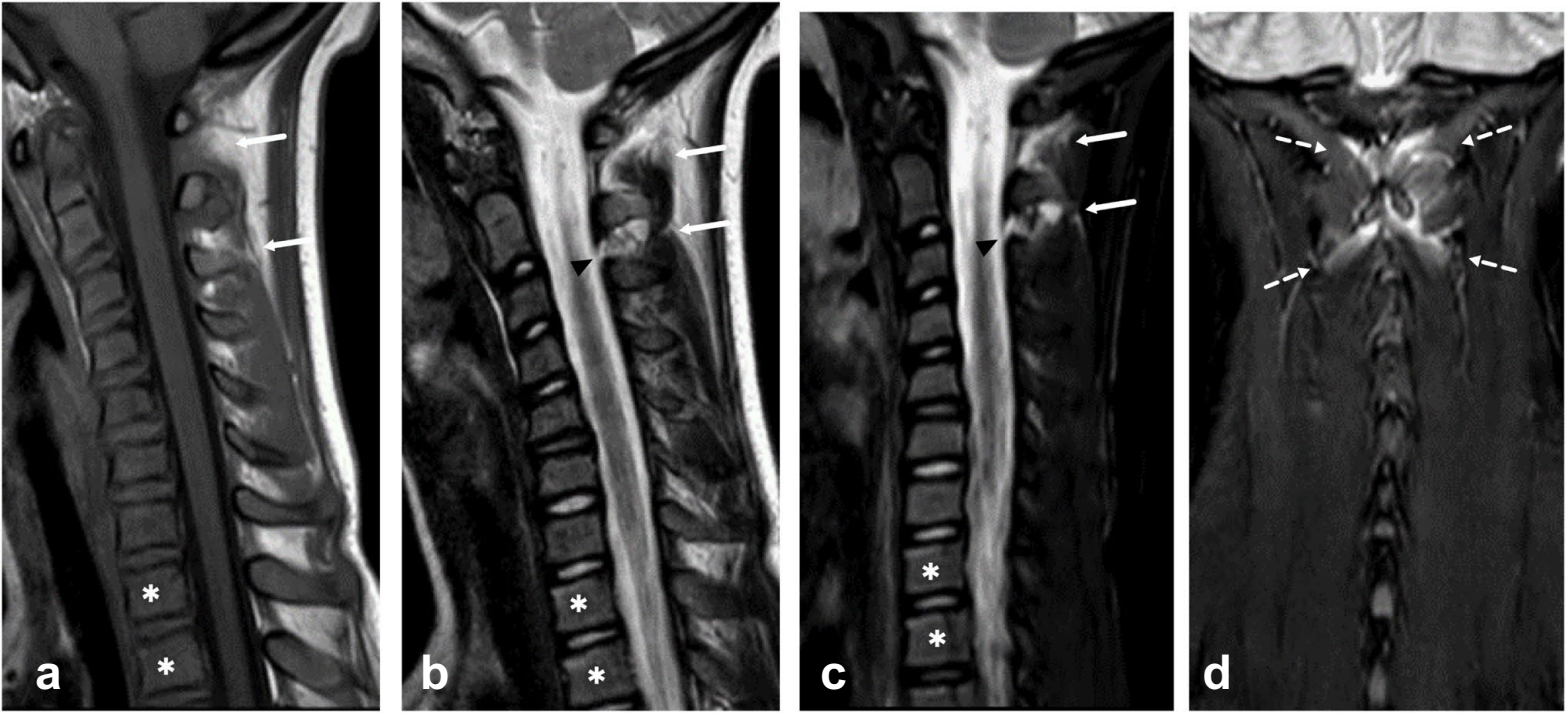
Images of a 10-year-old girl after a trampoline accident. Sagittal T1-weighted (a), sagittal T2-weighted (b), sagittal short tau inversion recovery (STIR) (c) and coronal STIR magnetic resonance images show posterior ligament complex injury at the cervical (C)1–C3 levels and minor compression fractures of thoracic vertebral bodies 1 and 2 (*asterisks*). The ligamentum flavum (*arrowheads*) is torn at C2/C3 and the interspinous and supraspinous ligaments (*arrows*, the two ligaments cannot be identified separately in these images) are torn at C1/C2 and C2/C3 levels. The suboccipital muscles are edematous (*broken arrows*)
